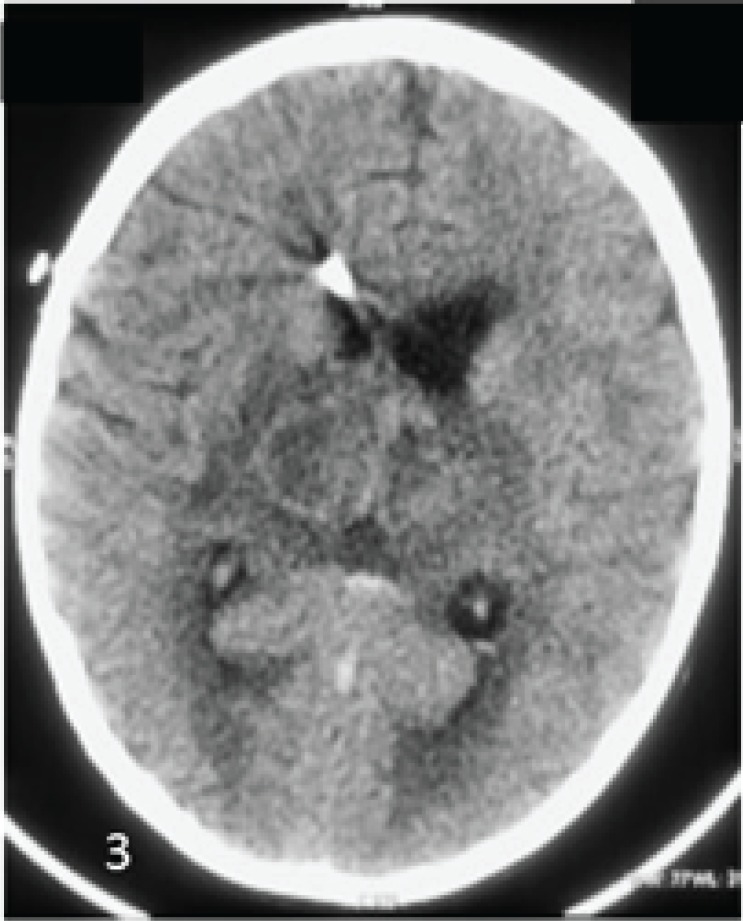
Axial non contrast CT post thrombolysis shows resolution of hyperdensity in the internal cerebral vein & the straight sinus. The thalamic hemorrhage is resolving. External ventricular drain is noted in the right frontal horn decompressing the ventricular system
